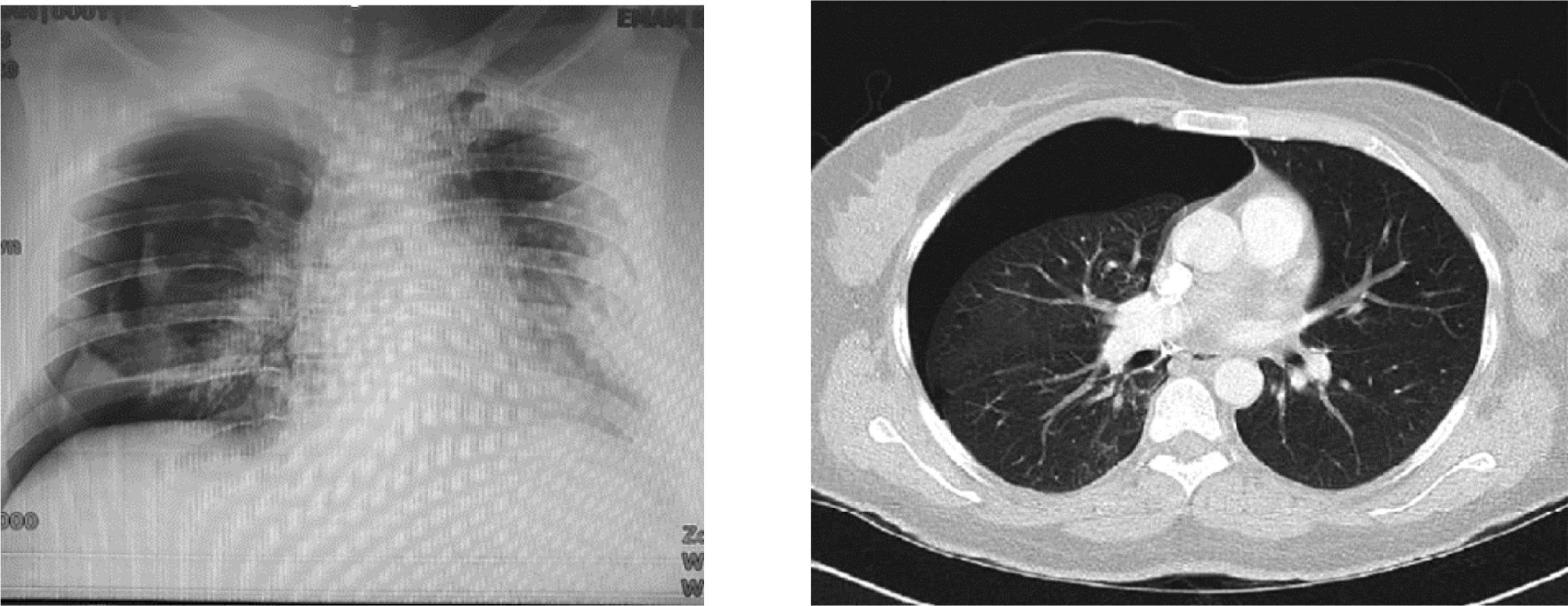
Patient's chest X‐ray and chest CT scan consisting of new right‐sided pneumothorax (late pneumothorax).
